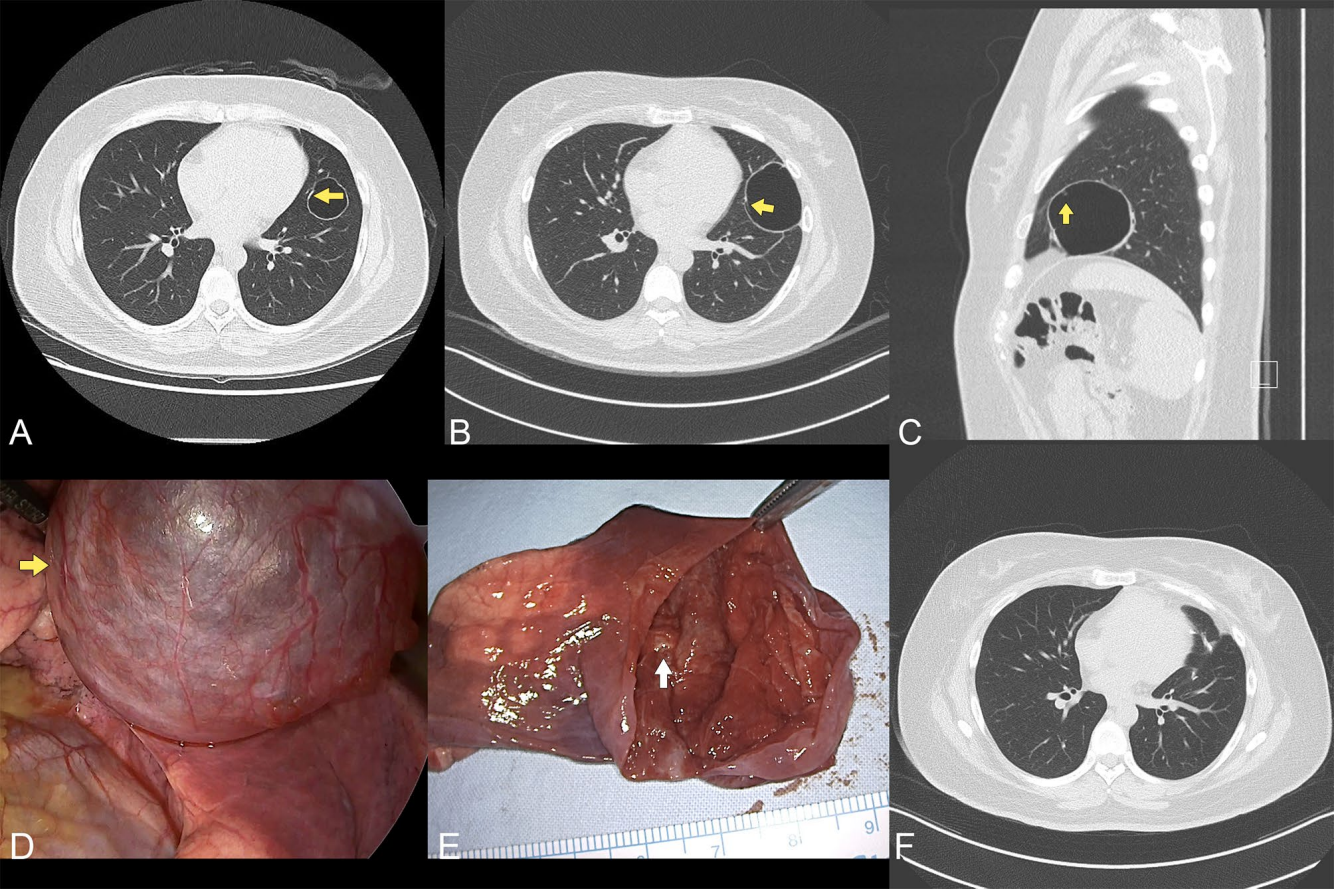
Diagnostic imaging and surgical findings: (A) initial chest CT demonstrating the 35-mm cyst; (B, C) axial and coronal views of cystic enlargement to 90 mm on subsequent CT. Yellow arrows indicate the suspected communication between cyst and bronchiole. (D) Intraoperative view of the cyst; (E) the opened cyst. The white arrow shows the terminal bronchiole, which has communication to the cyst. (F) Follow-up CT scan 2 years after surgery shows the healed surgical site
